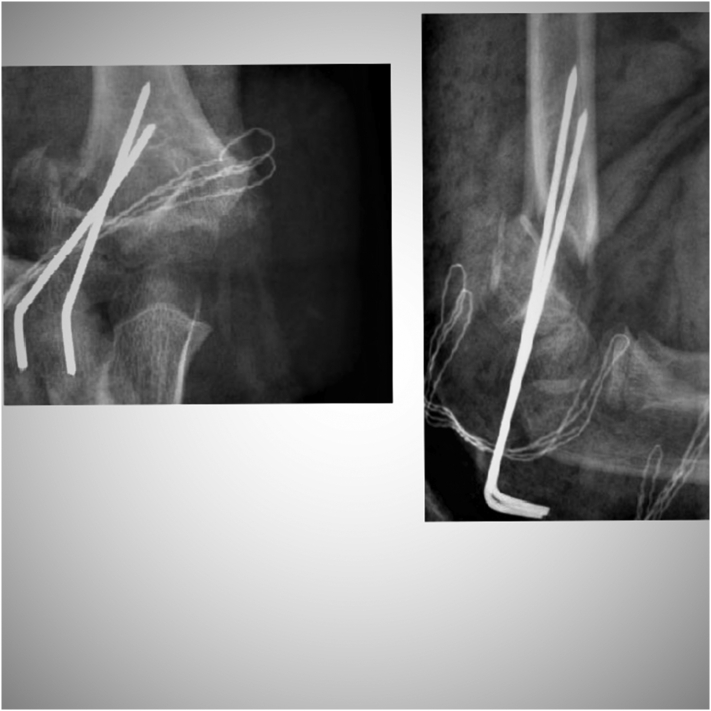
Intraoperative radiograph. Unsuccessful closed reduction and lateral pinning. AP and lateral view.
